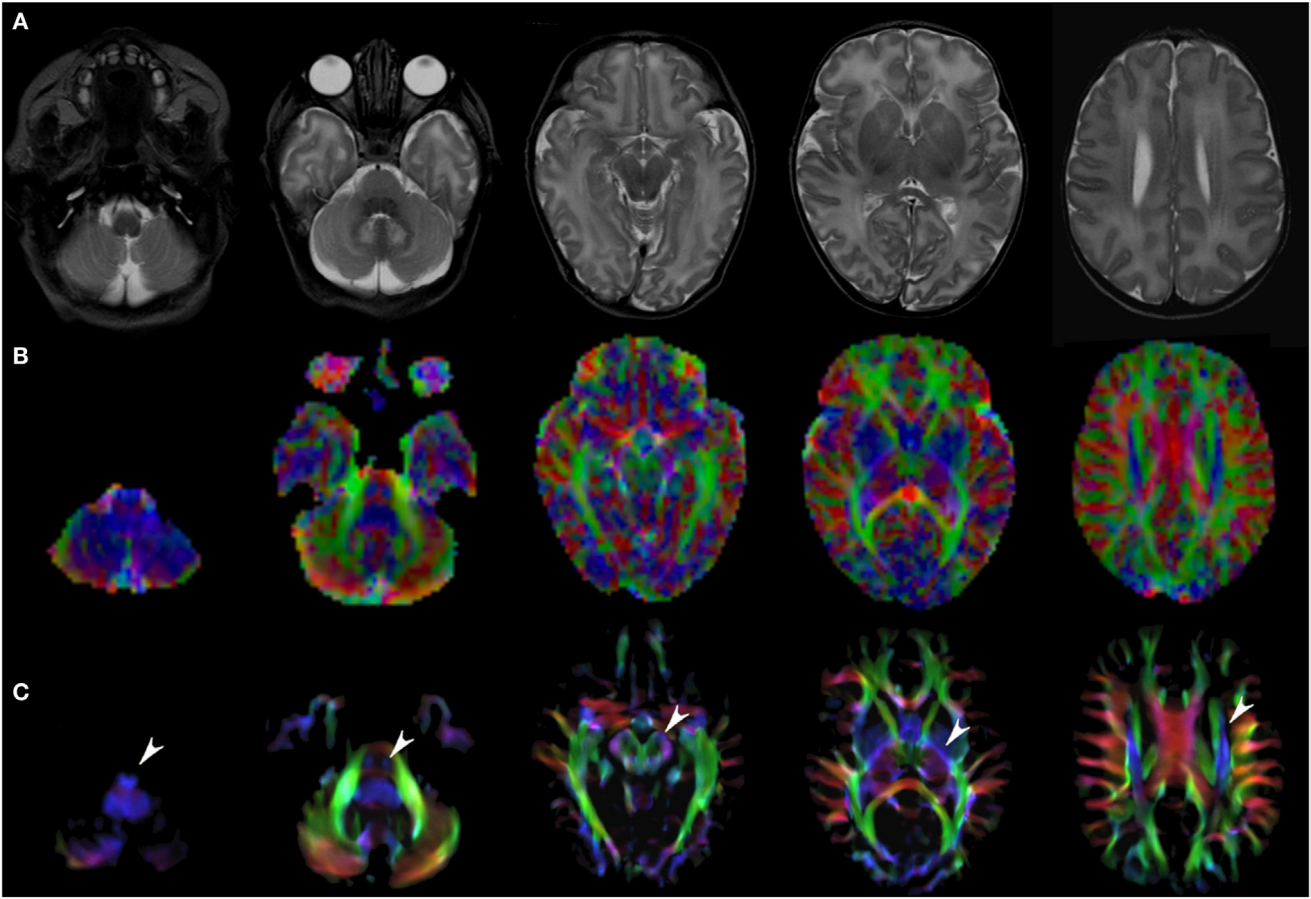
EV maps and short-track TDI maps at 5 canonical brain levels (rostral medulla, middle pons, rostral midbrain, internal capsules, and corpus callosum) in a 6-day neonate. Axial T2-weighted images ((A) upper row), corresponding EV maps ((B) middle row), and stDEC-TDI images ((C) bottom row). Conventional color scheme: blue (inferior–superior), green (anteroposterior), and red (left–right). Note that even in an unmyelinated brain the corticospinal tract is clearly visible from the bulbar level to the centrum semiovale in the stDEC-TDI map (arrowheads), while it is more difficult to distinguish in the EV maps especially at the bulbar level (first column).
